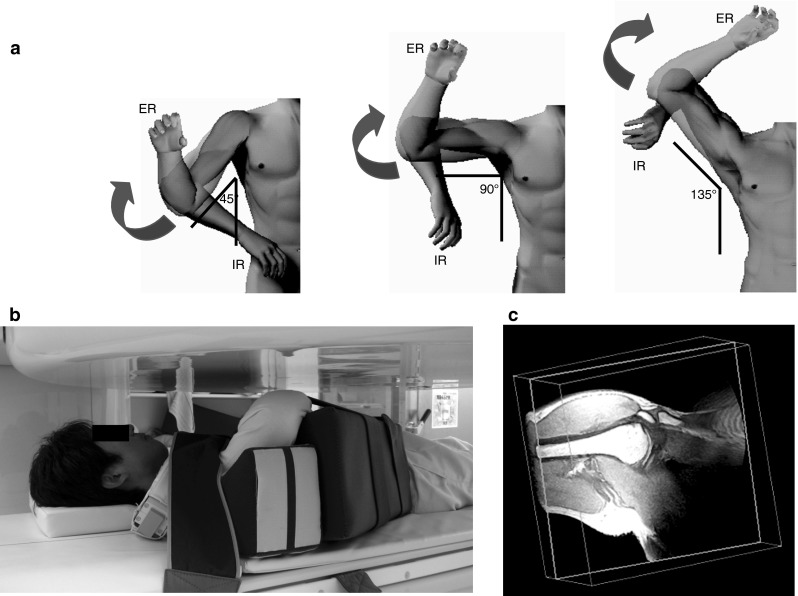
a The angle of the upper extremity in relation to the trunk was controlled to determine the six positions. b The volunteer was placed in an open magnetic resonance imaging system. The arm position was controlled by a positioning device. c A three-dimensional computer-generated magnetic resonance image
